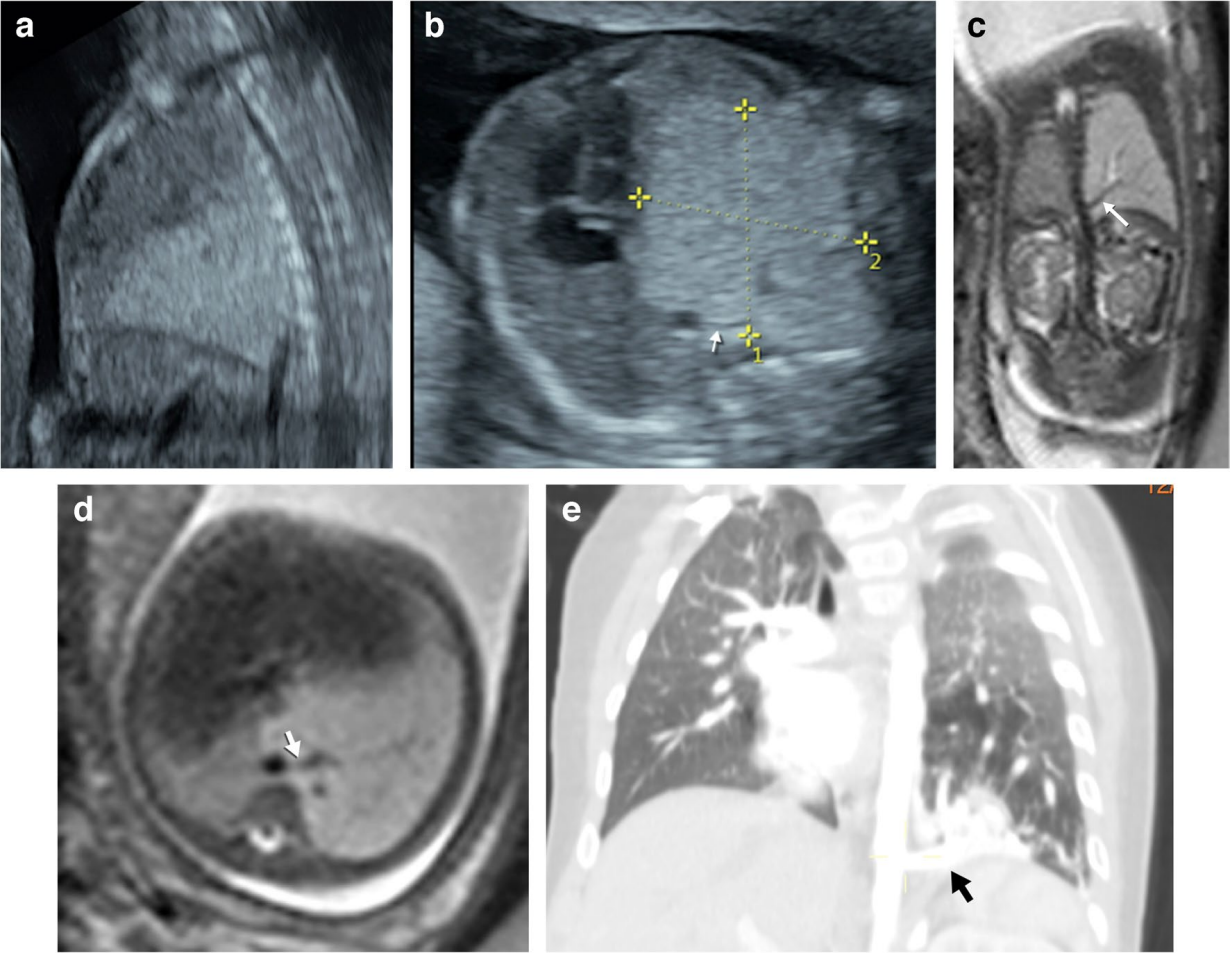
An intralobar bronchopulmonary sequestration in a 20-week gestational age female fetus. a, b Sagittal (a) and axial (b) ultrasound images demonstrate a hyperechoic lung lesion in the left lower lobe (*calipers* in b) mildly displacing the heart to the right with a linear hypoechoic structure entering it, suggestive of a feeding vessel (*arrow* in b). c, d Fetal magnetic resonance imaging was performed at 21 weeks of gestation. Single-shot T2 coronal (c) and axial (d) images confirm the presence of a large lesion in the left lung, with homogeneous high T2 signal and a feeding vessel arising from the descending thoracic aorta (*arrow* in d). e Contrast-enhanced coronal computed tomography image on lung window obtained at 6 months of age confirms the lesion in the left lung base and the feeding vessel  (*arrow*). Surgery demonstrated intralobar sequestration
